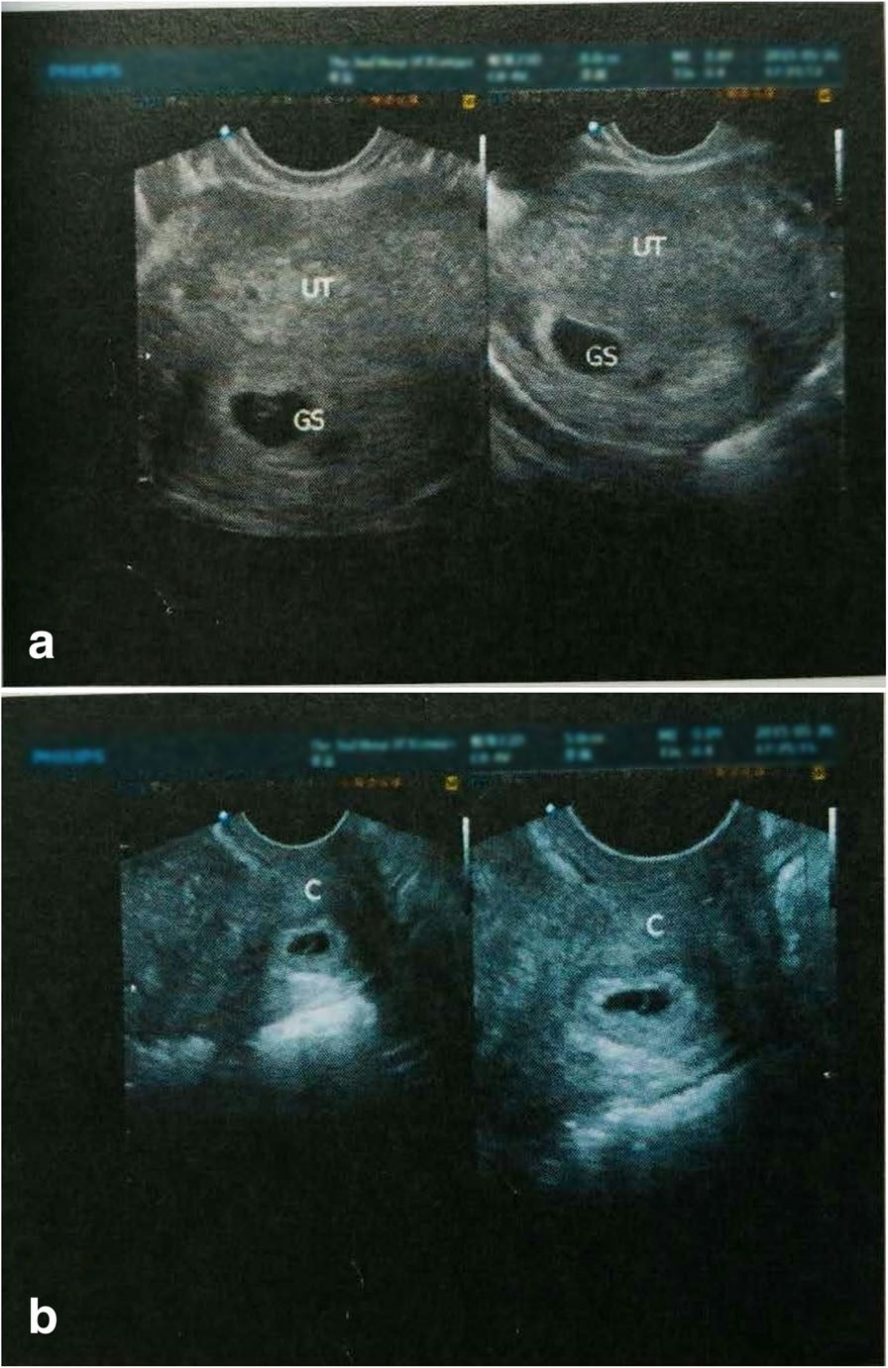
a Transvaginal Ultrasonography showing a gestational sac in the uterine cavity. GS = Gestational Sac, UT = Uterus. b Transvaginal Ultrasonography showing a gestational sac in the cervical canal. C = cervical canal.
